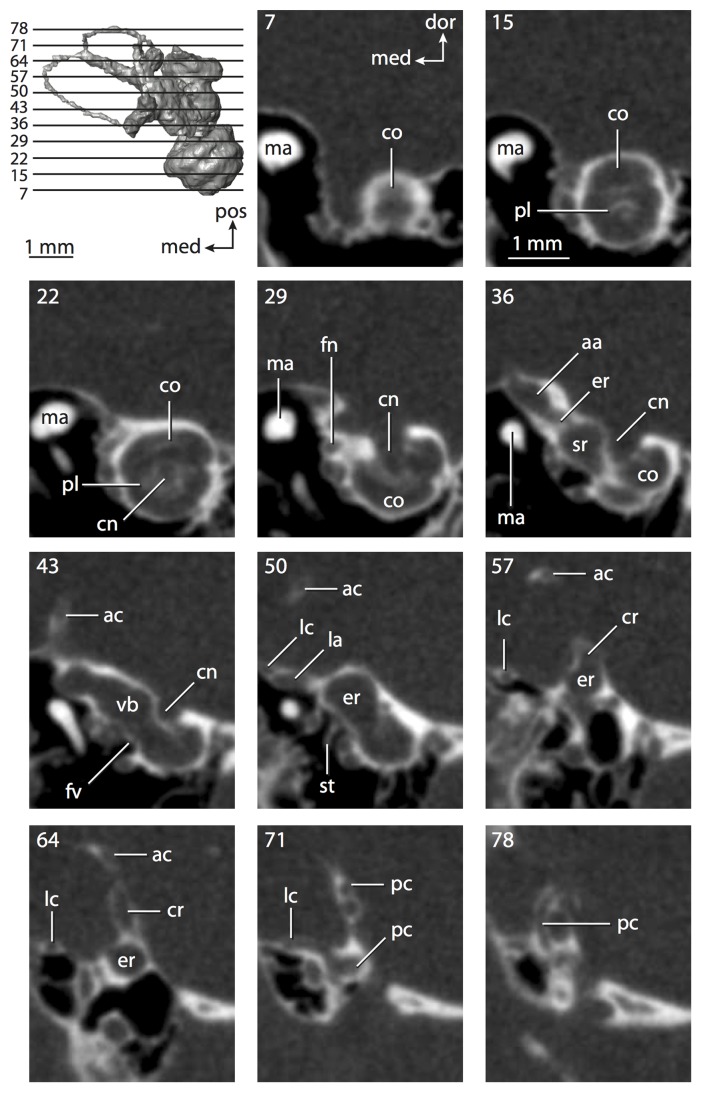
CT slices through ear region of*Chrysochloris* sp. Numbers refer to specific CT slices. Abbreviations listed at the end of the Materials and Methods section.
